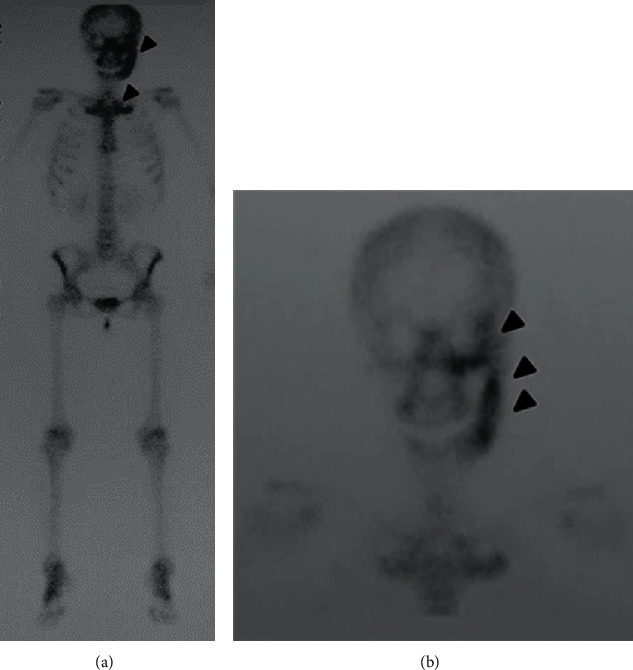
(a) Bone scintigraphy showing abnormal accumulation in the bilateral thoracic rib joints and sternum (arrowhead). It presents with the so-called bull's head sign. (b) Abnormal accumulation is observed in the temporal bone, cheekbone, sphenoid bone, and mandible (arrowhead).
